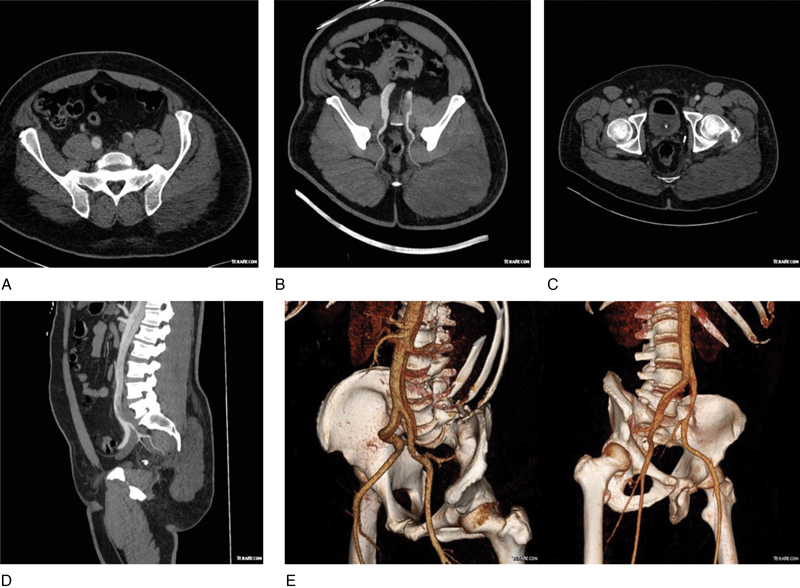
Computed tomography angiography prefasciotomy of maximum intensity projection. (
